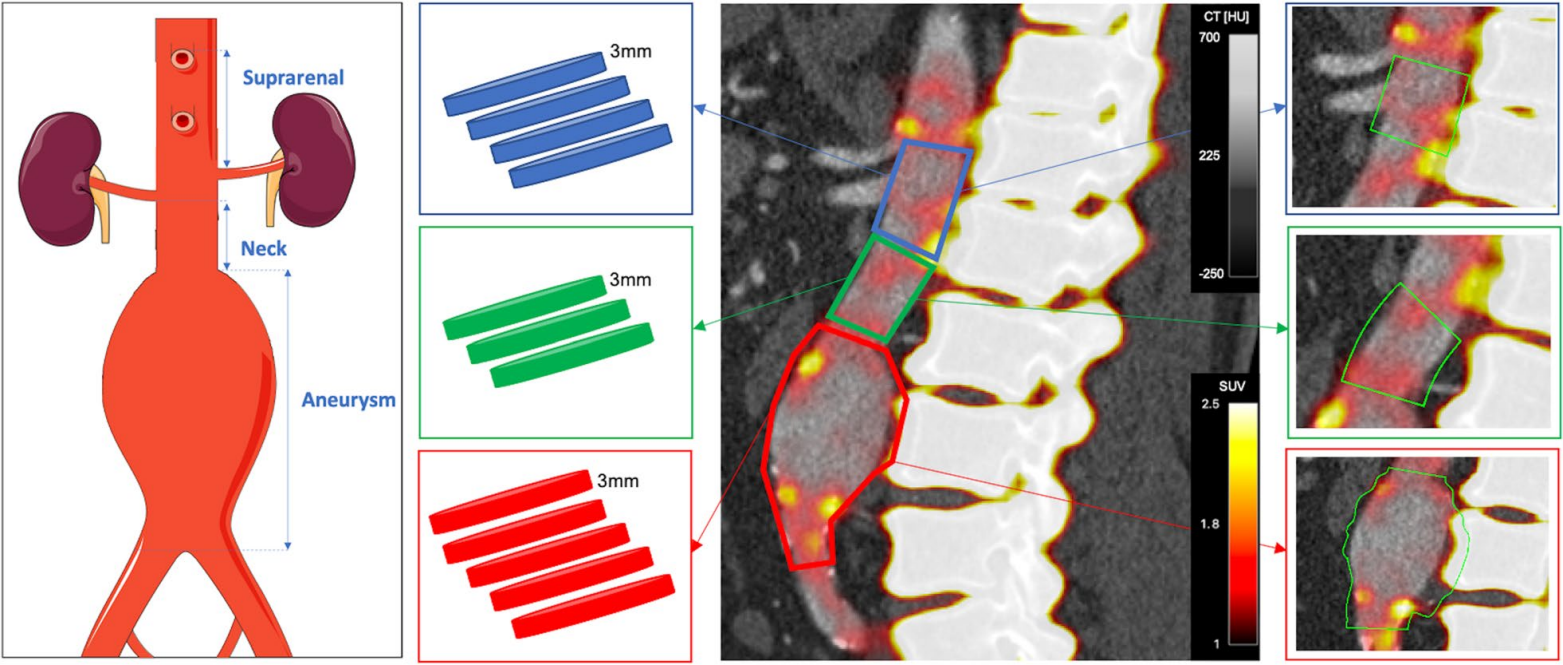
Regions of interest within the abdominal aorta. The abdominal aorta was split into three anatomical regions for analysis demonstrated on the panel on the left. Suprarenal—starting from the level of the origin of the coeliac artery till the upper-most renal artery. Neck—starting from the lower-most renal artery until where the aorta becomes aneurysmal. Aneurysm—starting just after the neck region ends until the aortic bifurcation. The second panel from the left shows a graphic representation of sequential 3 mm polygons taken from the three different aortic regions (suprarenal—blue, neck—green, aneurysm—red). The third panel from the left shows a sagittal view of the abdominal aorta. The right panel shows the volumes of interest drawn on the image analysis programme. CT [HU], computed tomography grey scale bar in Hounsfield units; SUV, positron emission tomography colour scale bar in standard uptake values
